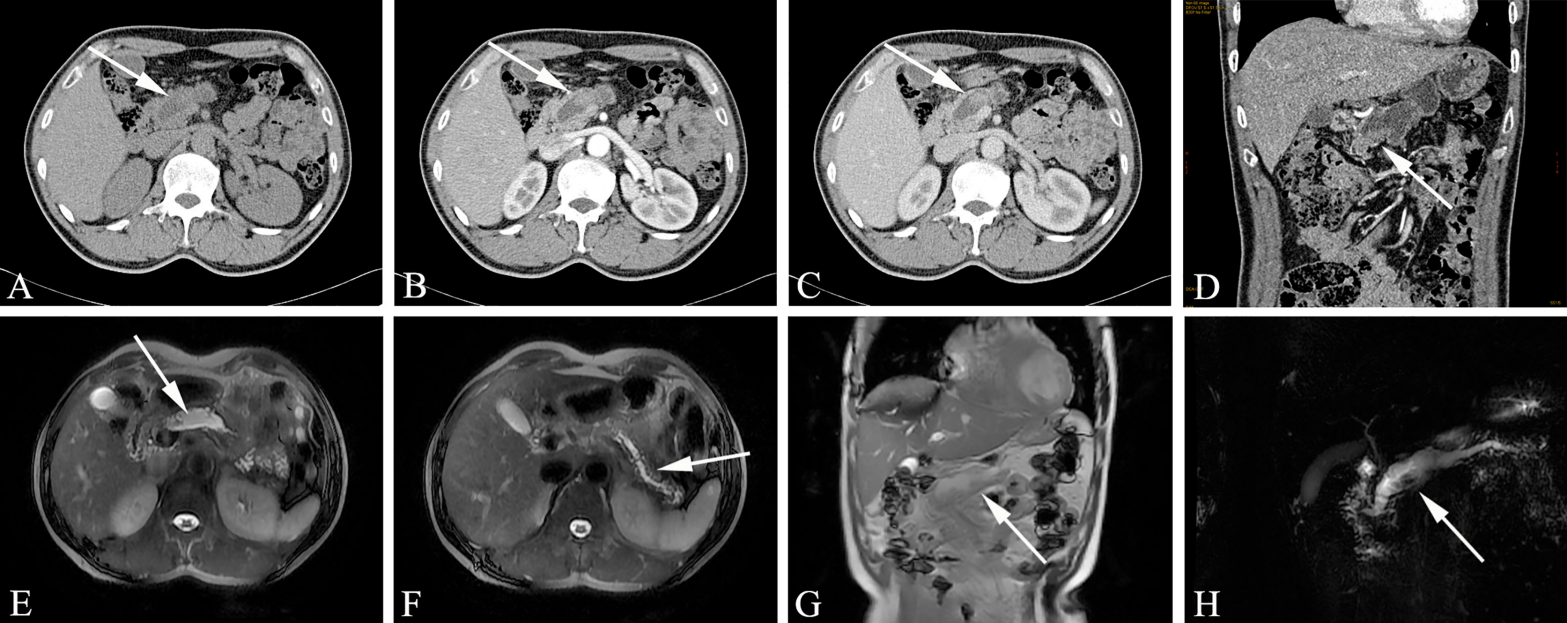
Main-duct IPMN (Male, 57y, abdomen pain for one month). The sequence distribution of images is the same as that in Figure 2. (A) The CT plain scan showed nodule in the neck of the pancreas, with a CT value of about 31HU. The MPD is obviously dilated, the pancreatic parenchyma is slightly atrophied, the dilated pancreatic duct is low-density, and the density is similar to that of water. (B, D) In the arterial phase, the nodule of the pancreatic neck was moderate enhanced, with a CT value of about 65HU, and the dilated pancreatic duct showed more clearly. (C) In the venous phase, the nodule showed continuous enhancement, at this time, the CT value is 72HU; (E–G) The T_2_-weighted imaging shows pancreatic duct dilatation with multiple mural nodules. (H) MRCP shows the MPD dilated significantly throughout the whole pancreas. The white arrows in the Figures only indicate the location of the neoplasm.
